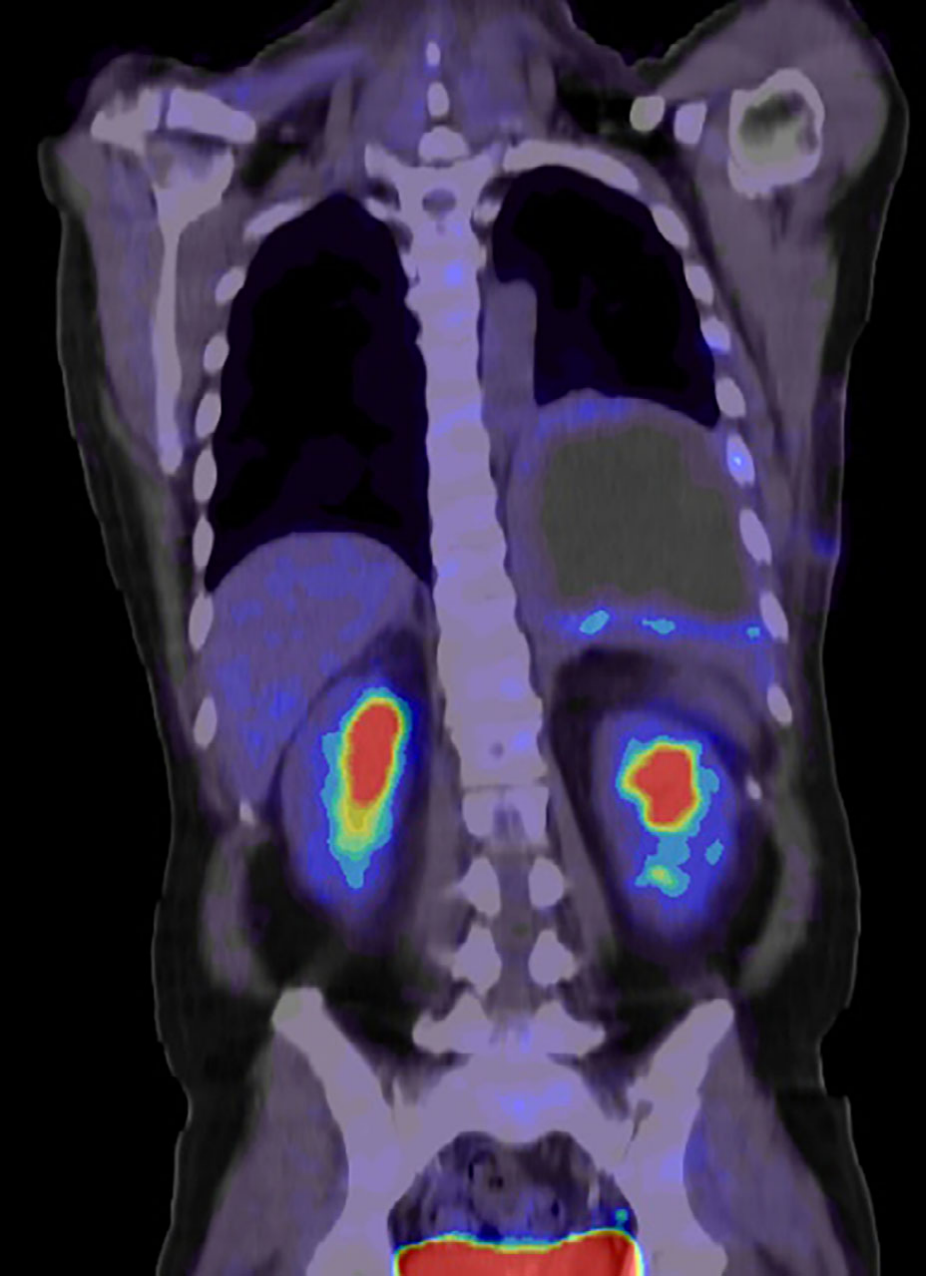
^18^F‐Fluorodeoxyglucose (FDG)‐positron emission tomography scan. FDG uptake was detected heterogeneously in the circumference of the mass. Standardized uptake value max was 5.5.
